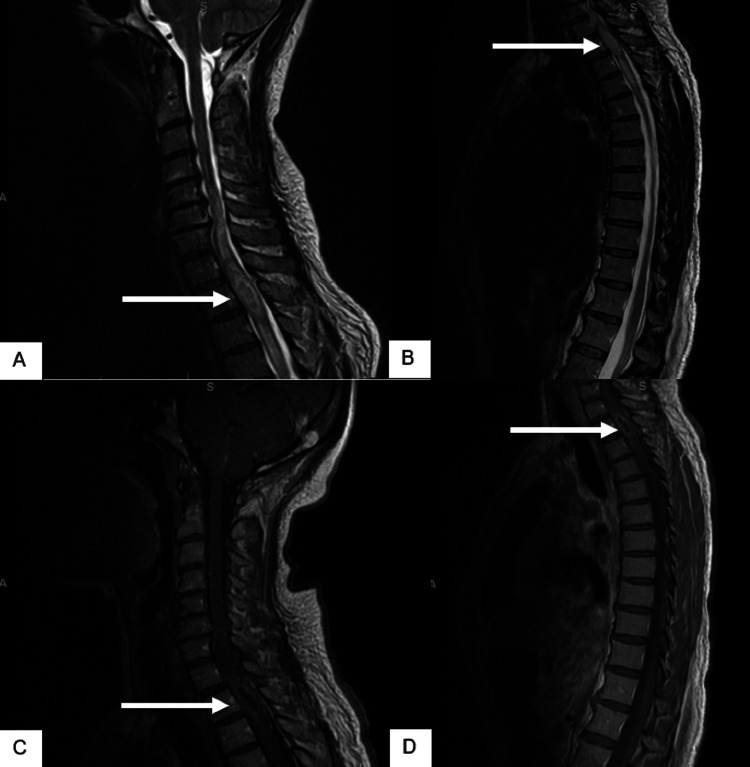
MRI of the cervicothoracic spine, sagittal view (A-B) T2-weighted and (C-D) T1-weighted post-contrast images showing an intradural lesion (white arrows) extending from the C5-T5 level.
